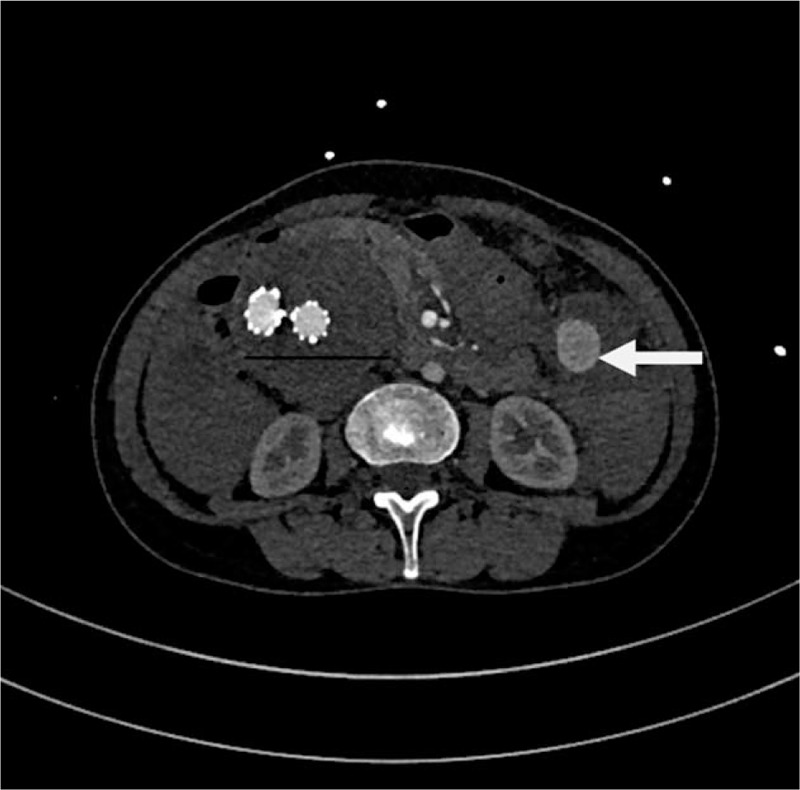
Preoperative computed tomography (CT) showing the aneurysm signs in the left abdomen, with effusion.
